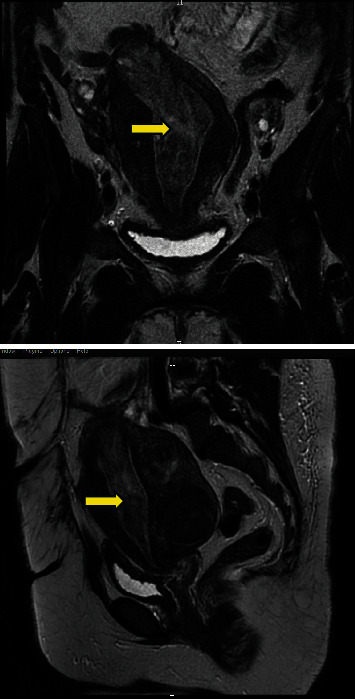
Pelvic MRI with gadolinium revealed an anteverted and bulky due to large fibroids within the posterior wall. Two intramural (FIGO 4) fibroids measuring 4.6 × 4.0 and 4.5 × 3.0 cm, respectively, were noted. Endometrium appeared thickened with a suspicion of a large pedunculated mass filling the whole uterine cavity, extending from the fundus to the internal cervical os.
